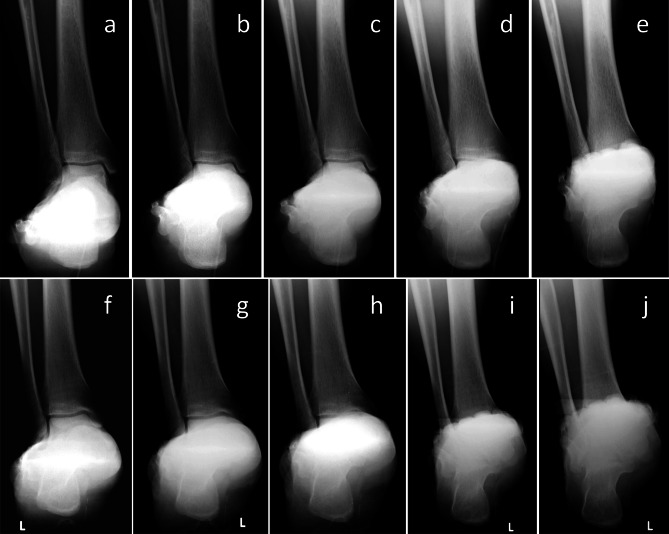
a-e X-ray scans of the hindfoot alignment position of normal volunteers at 10 degree, 15 degree, 20 degree, 25 degree and 30 degree. f-j X-ray scans of the hindfoot alignment position of patients with flatfoot at 10 degree, 15 degree, 20 degree, 25 degree and 30 degree
